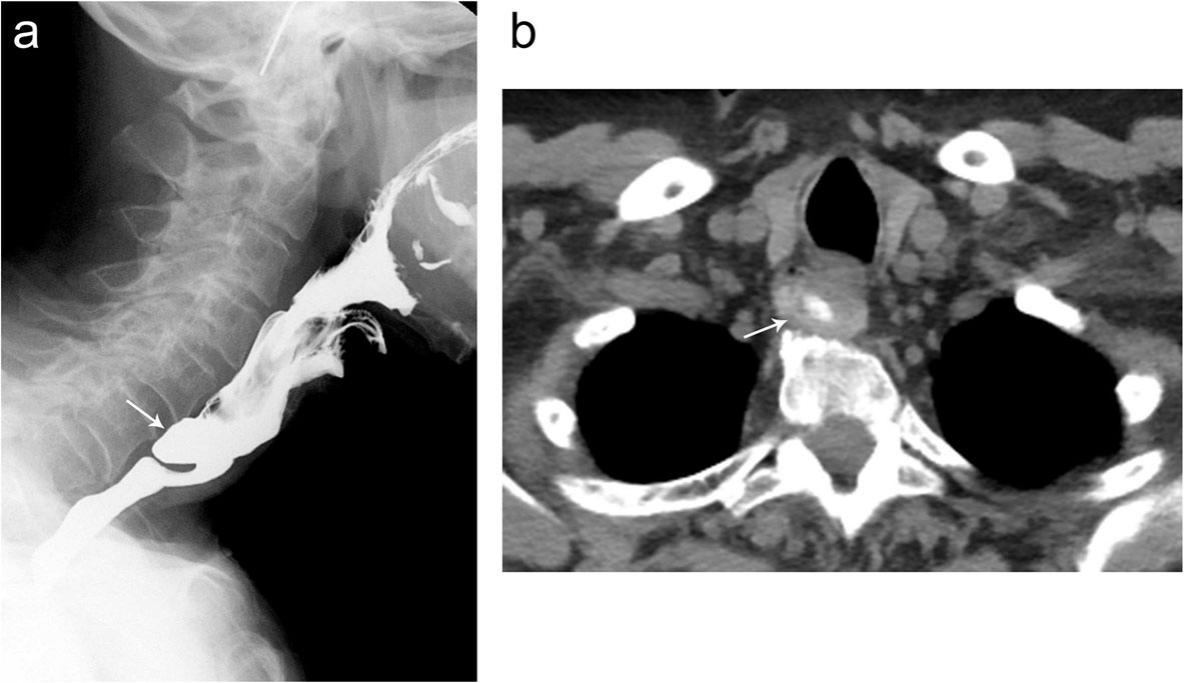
Zenker diverticulum. Lateral (a) fluoroscopic images demonstrate a focal outpouching from the posterior aspect of the esophagus containing an air-contrast level consistent with a Zenker diverticulum. Axial (b) noncontrast CT images from the same patient demonstrate residual oral contrast (arrow) within the Zenker diverticulum arising from the posterolateral aspect of the esophagus
